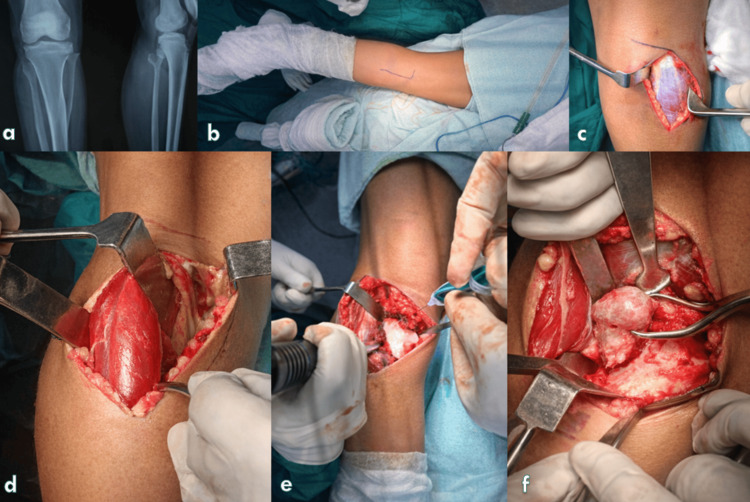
Stepwise surgical exposure of the posterior proximal tibia demonstrating posterior approach and muscle retraction (a) Preoperative anteroposterior and lateral radiographs of the knee.
